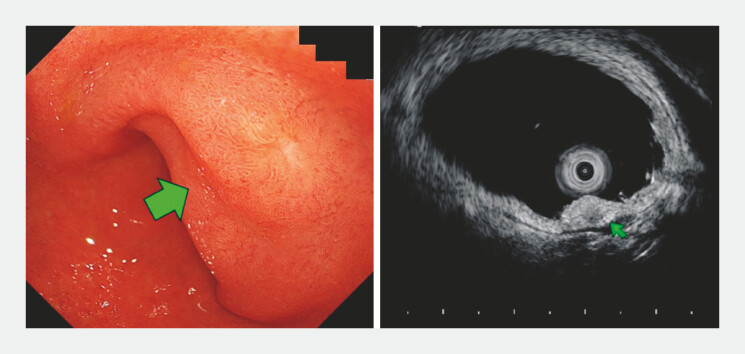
Endoscopic ultrasonography showing a submucosa-confined lesion without muscularis
